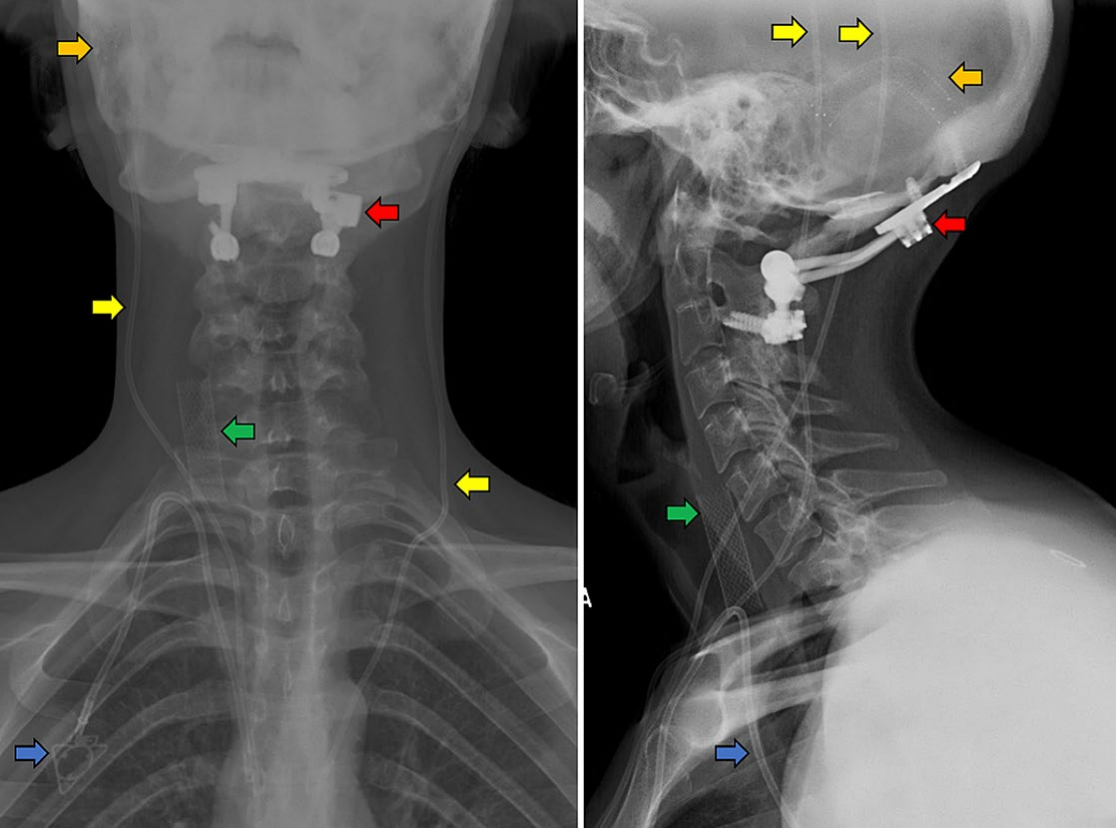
Anteroposterior (left panel) and lateral (right panel) cervical x-rays of an example patient with CVD and EDS. A Caucasian, tall, non-obese female in her 20’s with hypermobility and loose skin who has previously undergone transverse sinus stenting (orange arrows) and bilateral ventriculoperitoneal shunting (yellow) for IIH with associated CSF leaks, occipito-cervical fusion (red) for CCI, right IJV stenting (green) and port placement for fluid infusions for POTS (blue).
